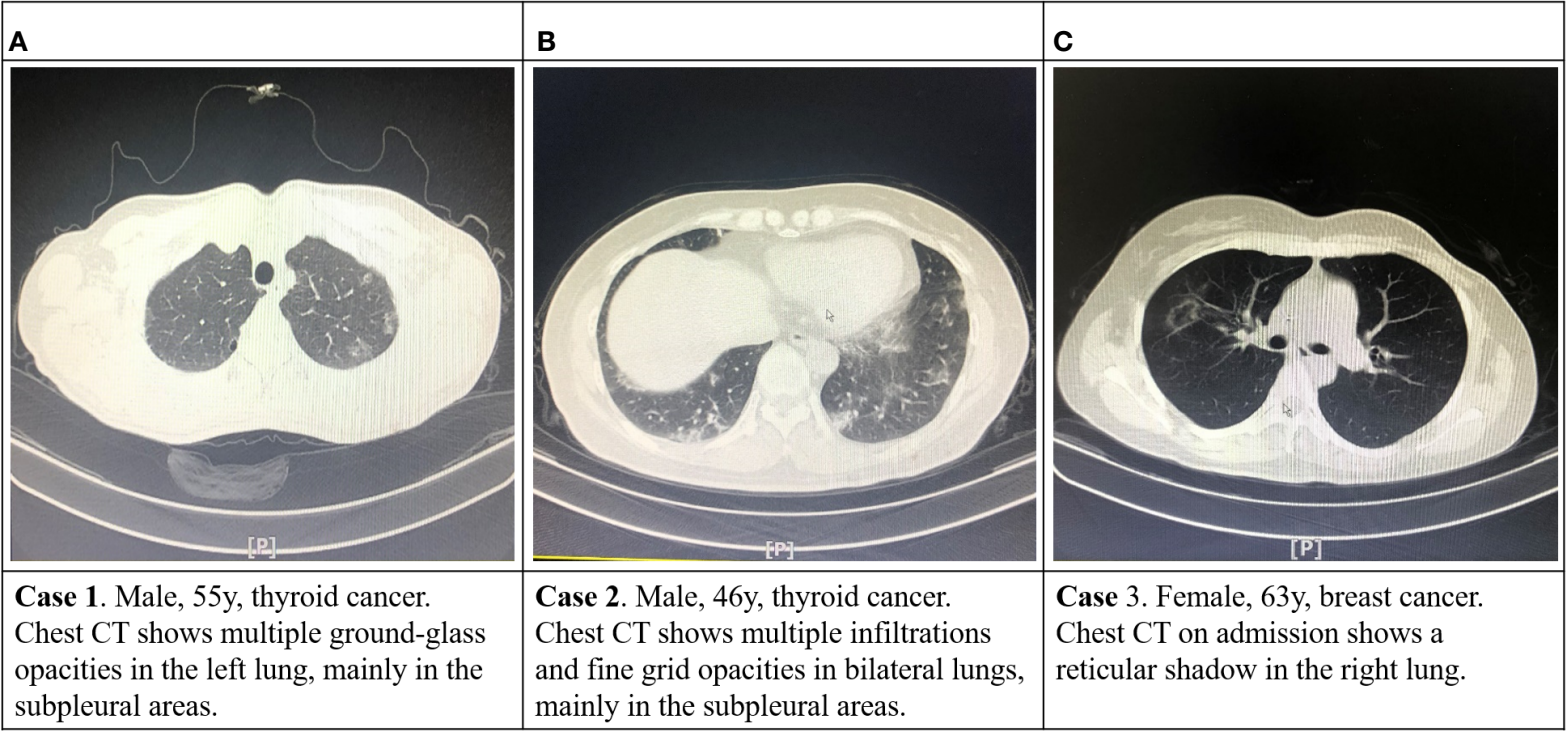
The chest CT images of three suspected asymptomatic NCP infection cases.
